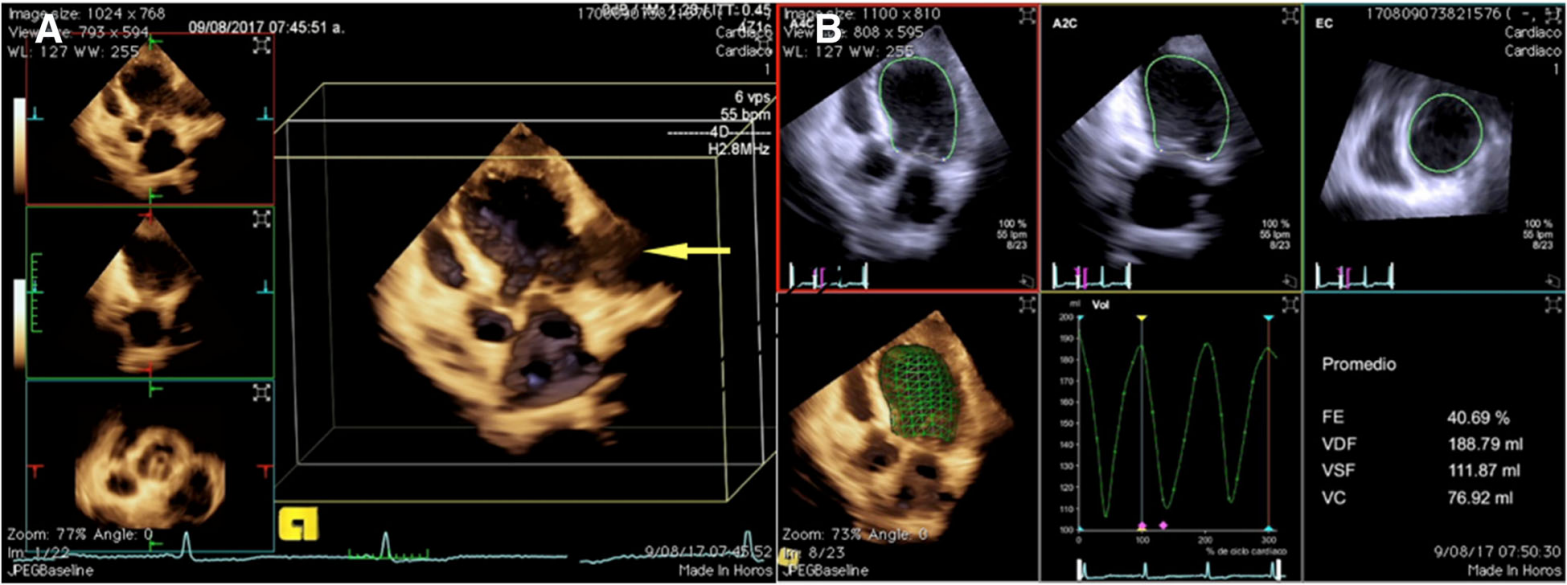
3D transthoracic echocardiogram showing a dilated left ventricular cavity and the PSA [arrow] (a) and the calculation of the LV end diastolic and systolic volumes, and LV ejection fraction (40%) by 3D method (b)
